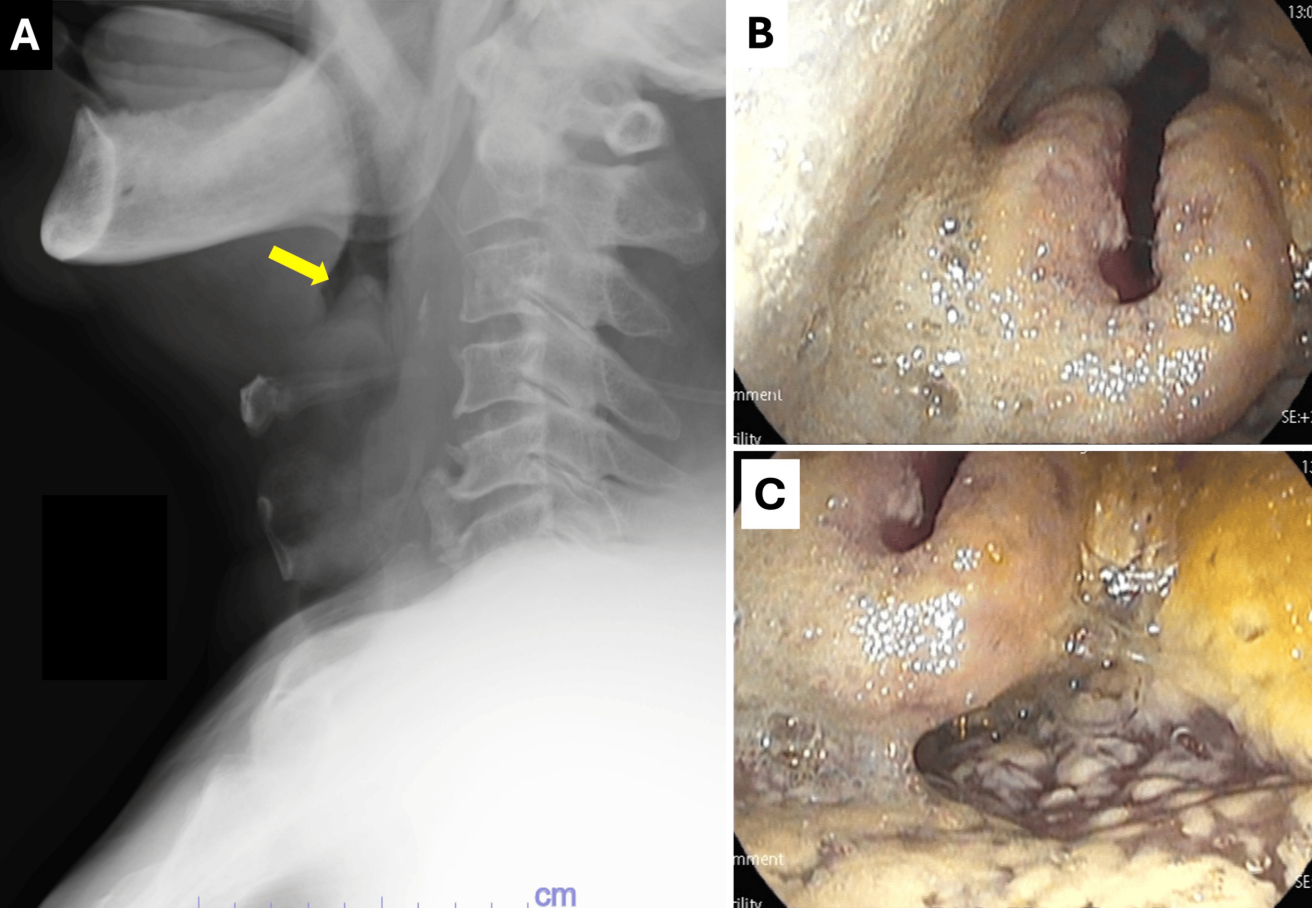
Diagnostic imaging and endoscopic findings of Candida epiglottitis (A) Lateral neck X-ray demonstrating swelling of the epiglottis, exhibiting the classic “thumb sign” characteristic of epiglottitis (indicated by an arrow). (B, C) Laryngoscopic views of the oropharynx revealing extensive white pseudomembranous lesions, along with swelling of the epiglottis and surrounding tissues, consistent with Candida epiglottitis.
